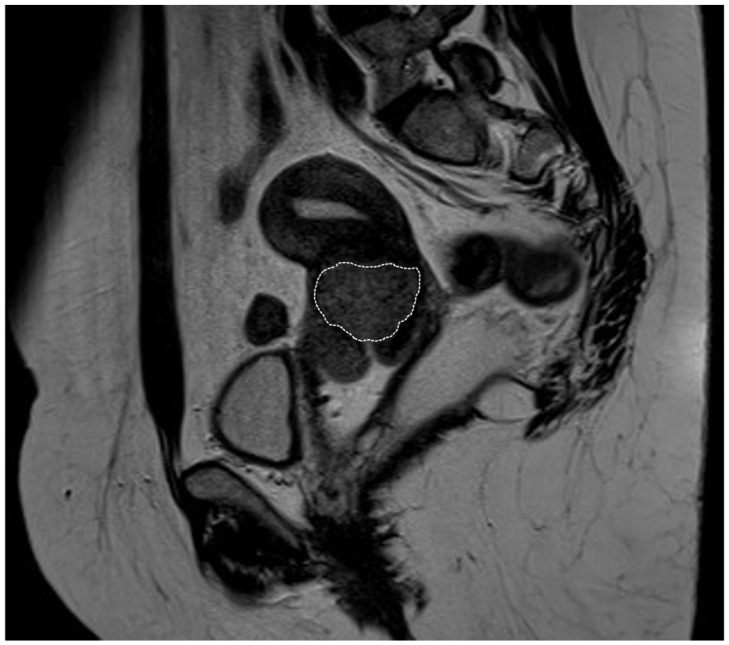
Pelvic magnetic resonance imaging of a bulky cervical tumor (marked with the dotted circle). The image corresponds to a 43-year-old patient diagnosed with cervical squamous cell carcinoma, with tumor dimensions of 43 × 41 × 39 mm on imaging and no parametrial involvement. She underwent primary surgical treatment with bilateral pelvic lymphadenectomy and radical hysterectomy (in 2015), with a postoperative stage of IIA2 (FIGO 2018). Adjuvant treatment with external beam radiotherapy (EBRT), chemotherapy (CT), and brachytherapy (BT) was administered. She was disease-free at the time of writing this article.
